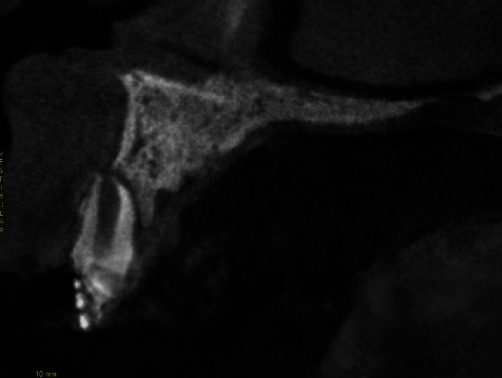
CT scan of tooth 11 area, where it is possible to note external root resorption and extensive resorption of the buccal wall.
